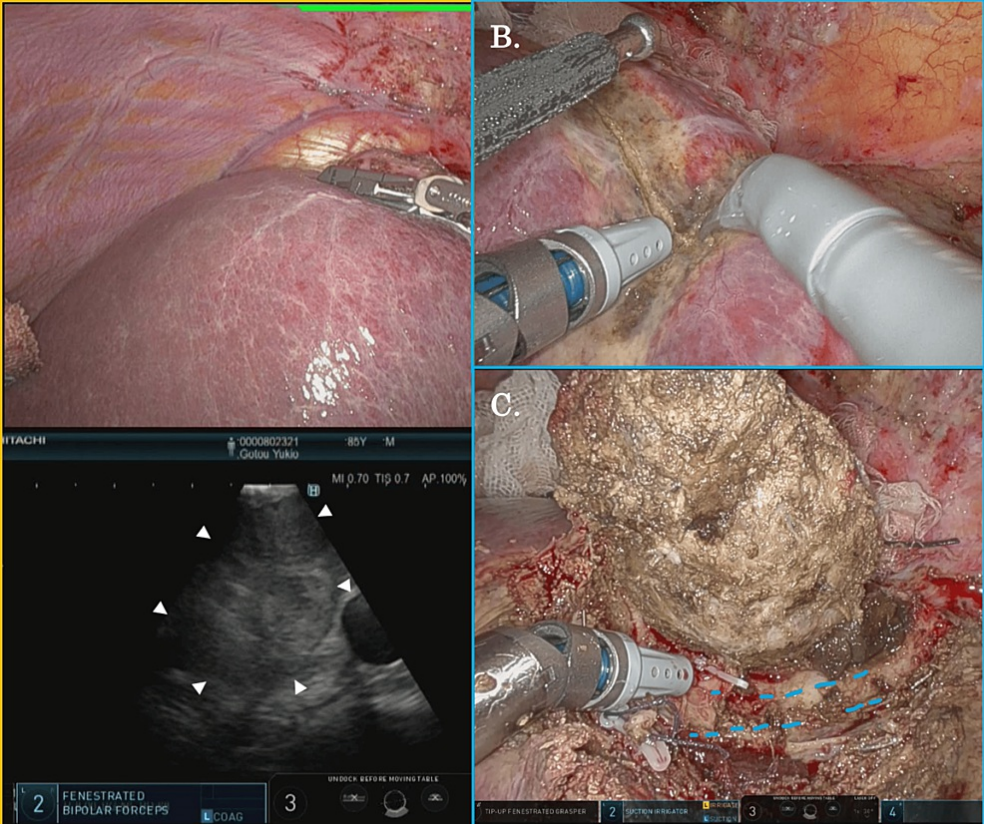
Operative findings during robotic S8vent anatomical liver resection. (A) An intraoperative ultrasound identified a tumor in liver S8 (arrows indicate the tumor). (B) Liver parenchymal transection using saline-linked monopolar scissors was performed during robotic liver resection. (C) Hepatectomy was completed while preserving both the middle and right hepatic veins (blue dotted lines).
